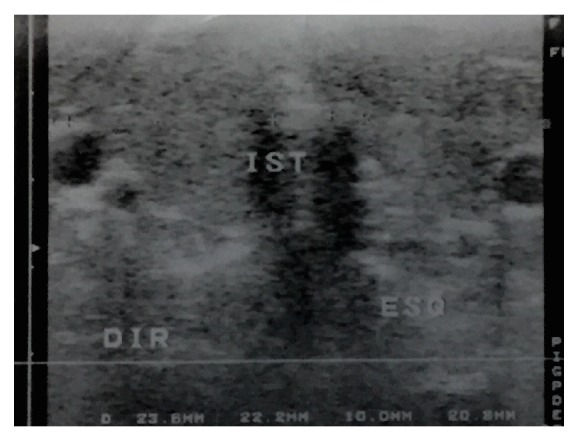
Ultrasound before thyroidectomy shows heterogeneous parenchyma with an estimated size of 45 cm^3^.
